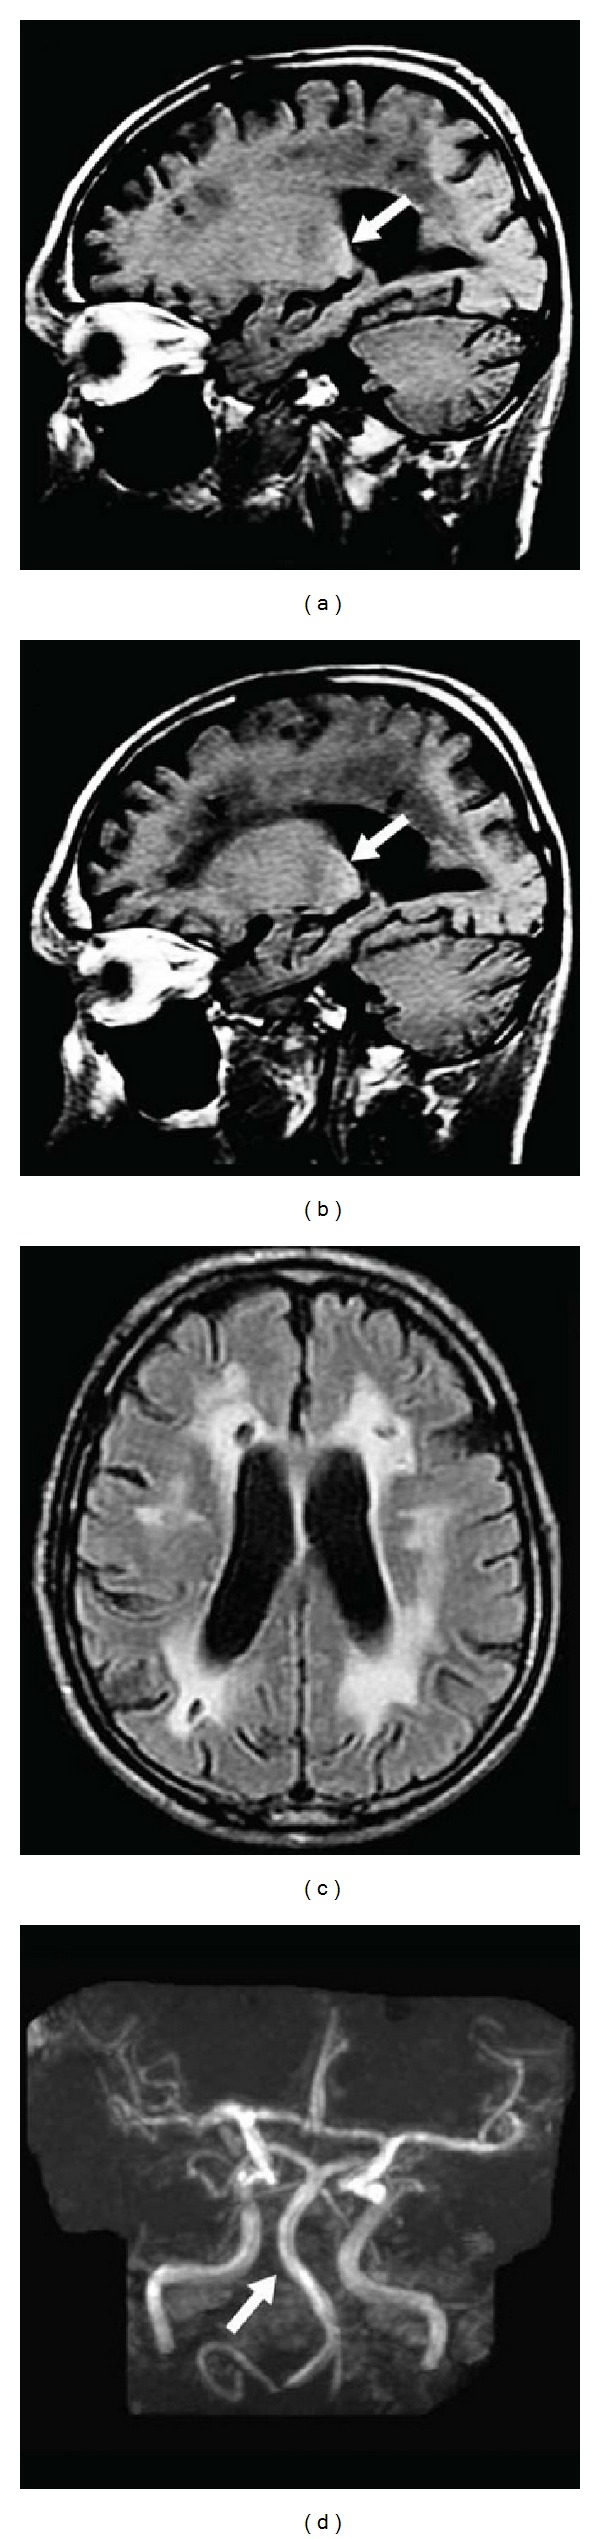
Sagittal unenhanced T1-weighted imaging (a and b) in a Fabry patient showing hyperintensity in the pulvinar, associated with ischemic leukoencephalopathy on FLAIR sequences (c) and vertebrobasilar dolichoectasia on TOF imaging (d).
